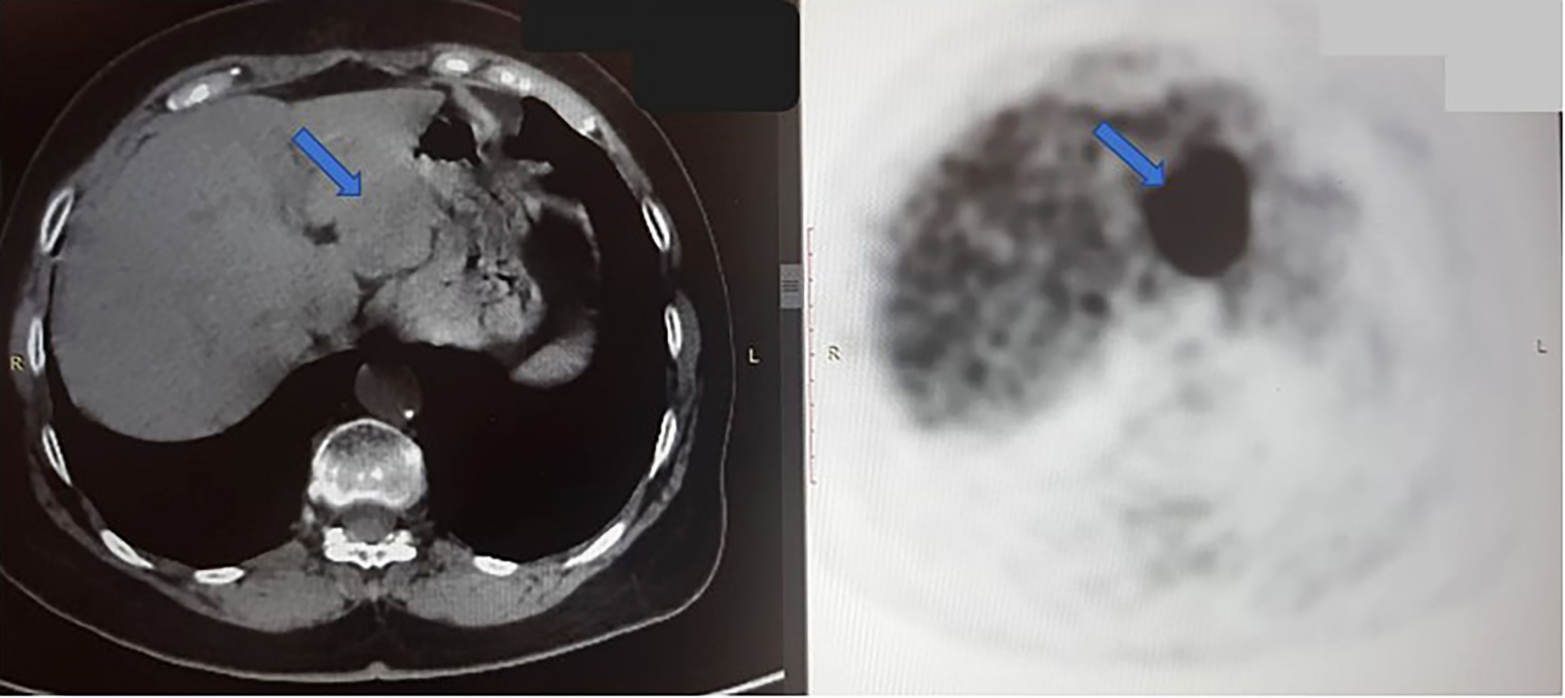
Axial CT scan (left) and PET scan (right) from October 2020, shows a large tumor in the left hepatic lobe (blue arrow). In PET scan, the dark mass shows an FDG-avid, metabolically active tumor. The right hepatic lobe is normal, without signs of malignancy at this time.
